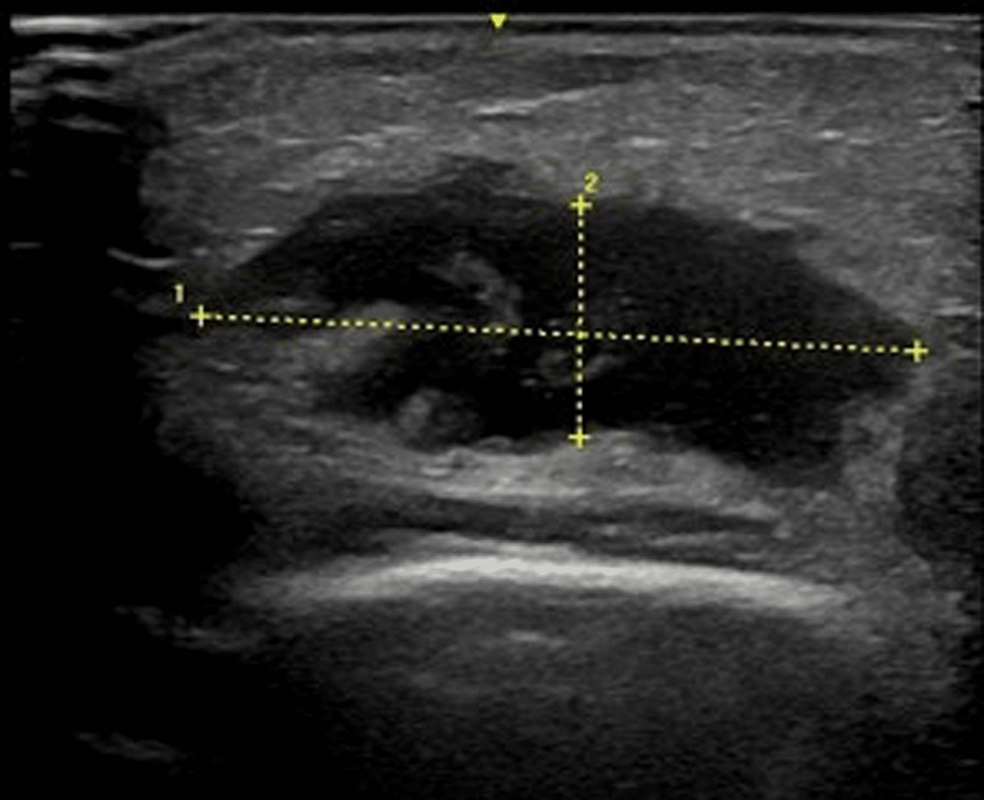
An ultrasound image of the left buccal region on the first visit A low echo area is seen (28.3 × 9.2 × 14.9 mm)
